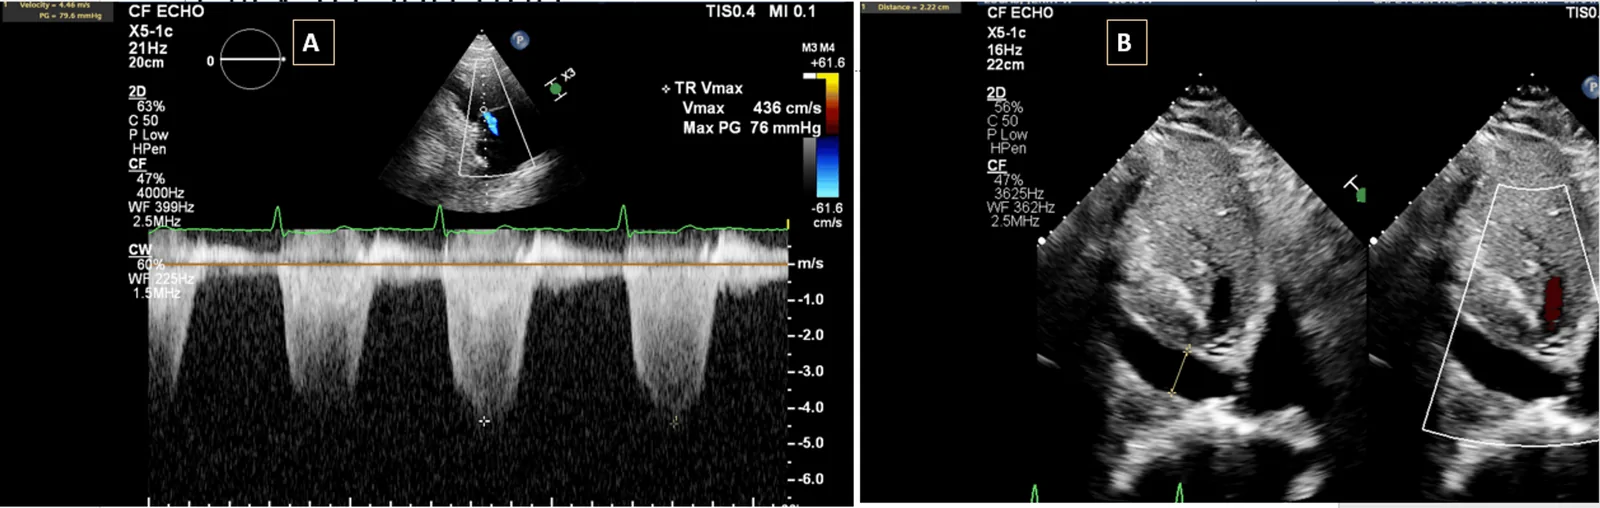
Follow-up echocardiographic assessment of right ventricular systolic pressure (A) Continuous-wave Doppler of the tricuspid regurgitation jet used for estimation of right ventricular systolic pressure (RVSP). The formal transthoracic echocardiographic interpretation reported a peak tricuspid regurgitation velocity of 4.39 m/s. (B) Subcostal view demonstrating a dilated inferior vena cava (IVC) measuring 2.22 cm. Based on IVC size and respiratory collapsibility, the formal echocardiographic interpretation assumed a right atrial pressure of 10 mmHg, yielding an estimated RVSP of 87.1 mmHg, consistent with severe pulmonary hypertension.
